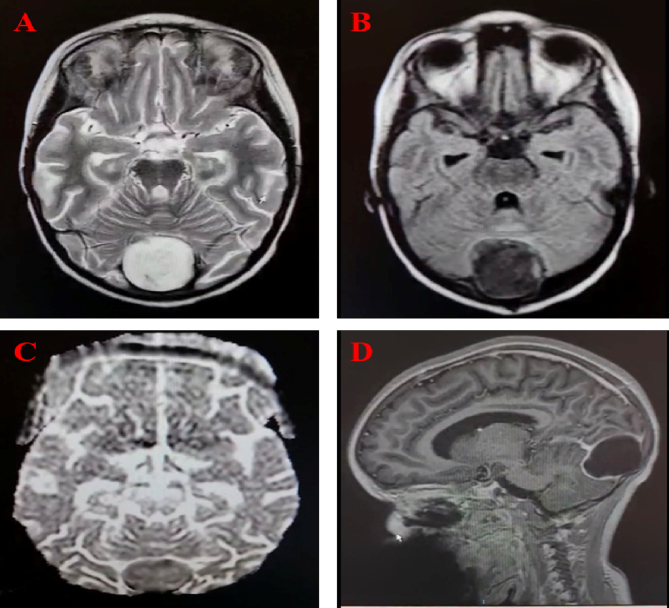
A-D: MRI of the brain (case 4) show a well circumscribed cystic lesion in the posterior fossa which causes splaying of the superior sagittal sinus and transverse sinuses bilaterally.
